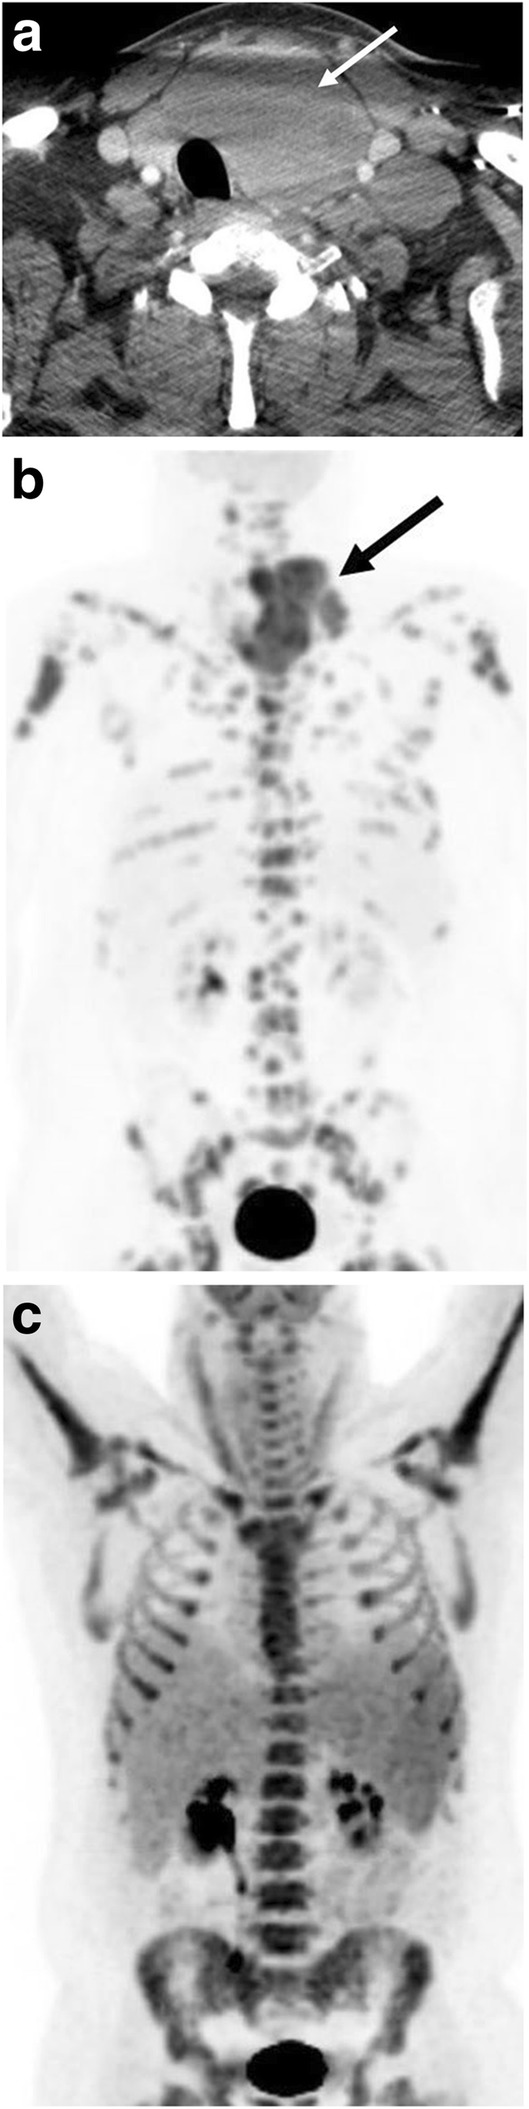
Treatment response. A 42-year-old man initially presenting with neck mass and constitutional symptoms. Contrast-enhanced axial CT at presentation (a) demonstrates a large left neck mass (arrow) replacing the left thyroid with tracheal deviation. b Staging whole body planar PET image demonstrates a hypermetabolic left lower neck mass (arrow) and heterogeneous marrow involvement. The patient underwent R-CHOP with intrathecal methotrexate, 4 cycles of hyper-CVAD and intrathecal methotrexate. Follow-up (c) whole-body planar PET image imaging show resolution of the cervical mass without evidence of distant disease. Diffuse homogeneous marrow uptake on the PET scan is likely related to post-therapy marrow activation
